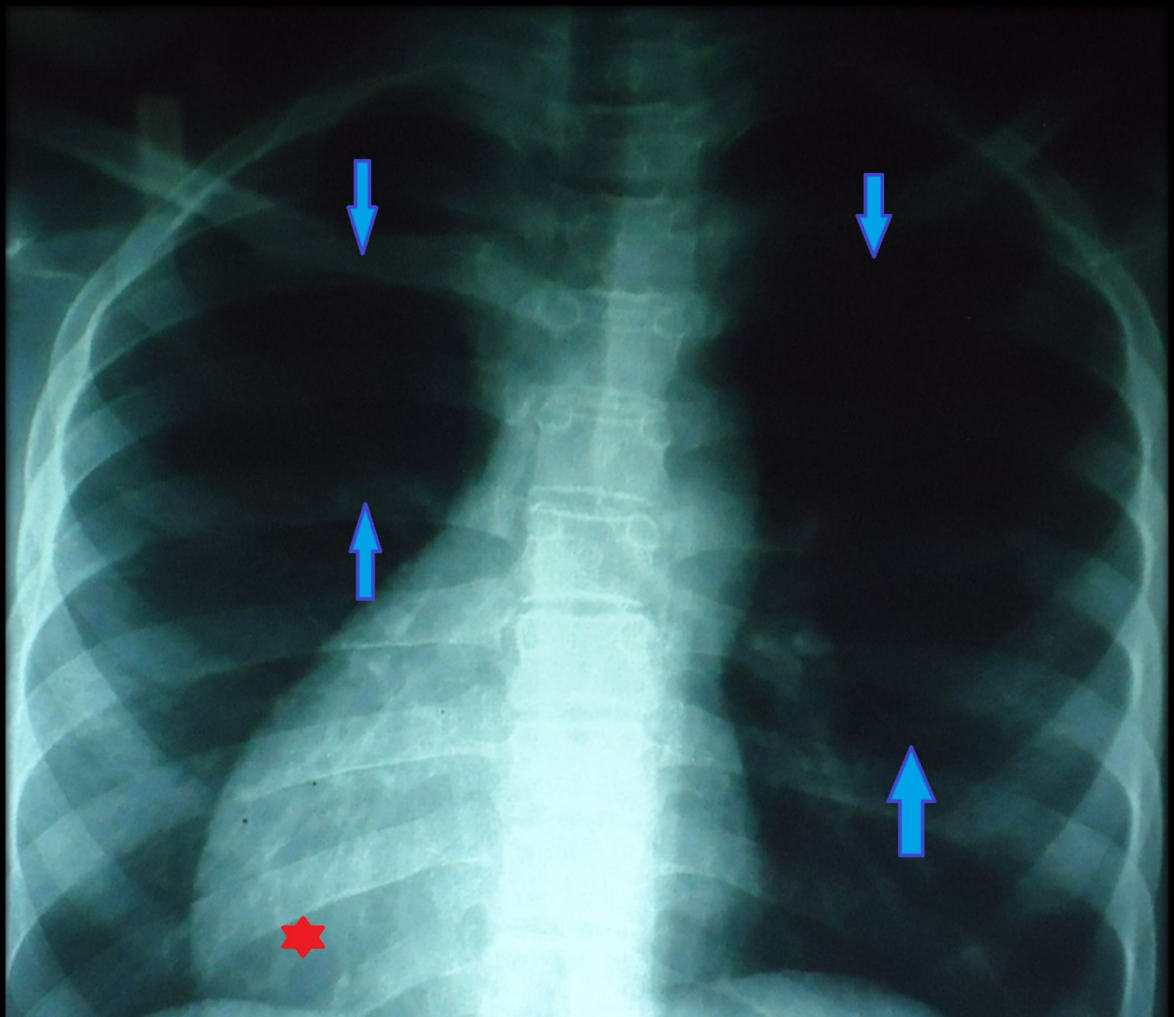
Chest X-ray PA view showing oligemic lung fields (shown in between the arrows) and dextrocardia (*) shown as inverted position of apex of heart towards the right side PA, posteroanterior
